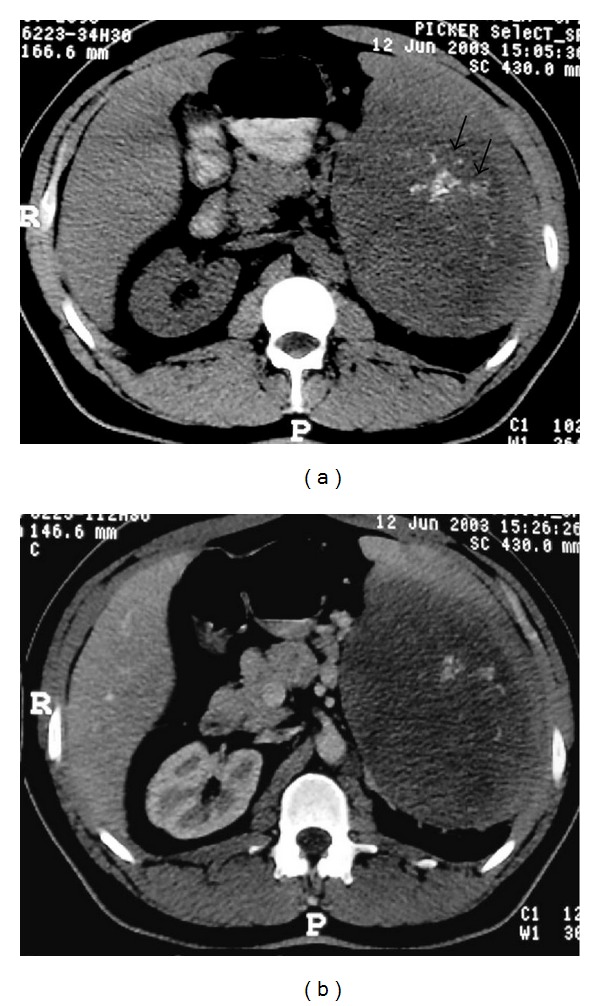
A 29-year-old man with NHL involving the spleen. Axial abdominal CT before (a) and after administration of intravenous CM (b) showing a large mass in the spleen (long arrows) with calcifications (small arrows).
